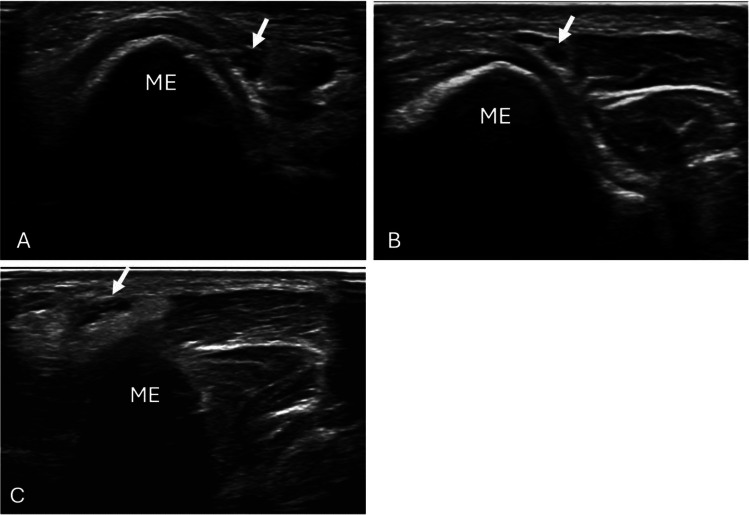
Axial ultrasound images of the medial elbow in a 16-year-old male football player with a snapping sensation and tingling at the medial elbow. Images obtained at approximately 15° (A), 30° (B), and 45° (C) of elbow flexion demonstrate anterior translation of the ulnar nerve (*arrows*) from posterior to the medial epicondyle (*ME*), confirming dynamic instability. The ulnar nerve shows abnormal morphology with fascicular enlargement and decreased echogenicity
